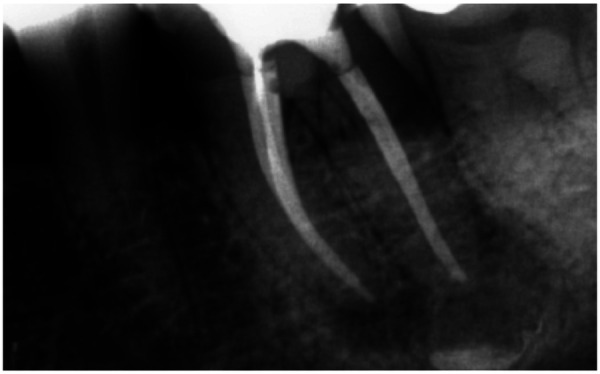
Left post-filling intraoral radiograph of tooth 36.
